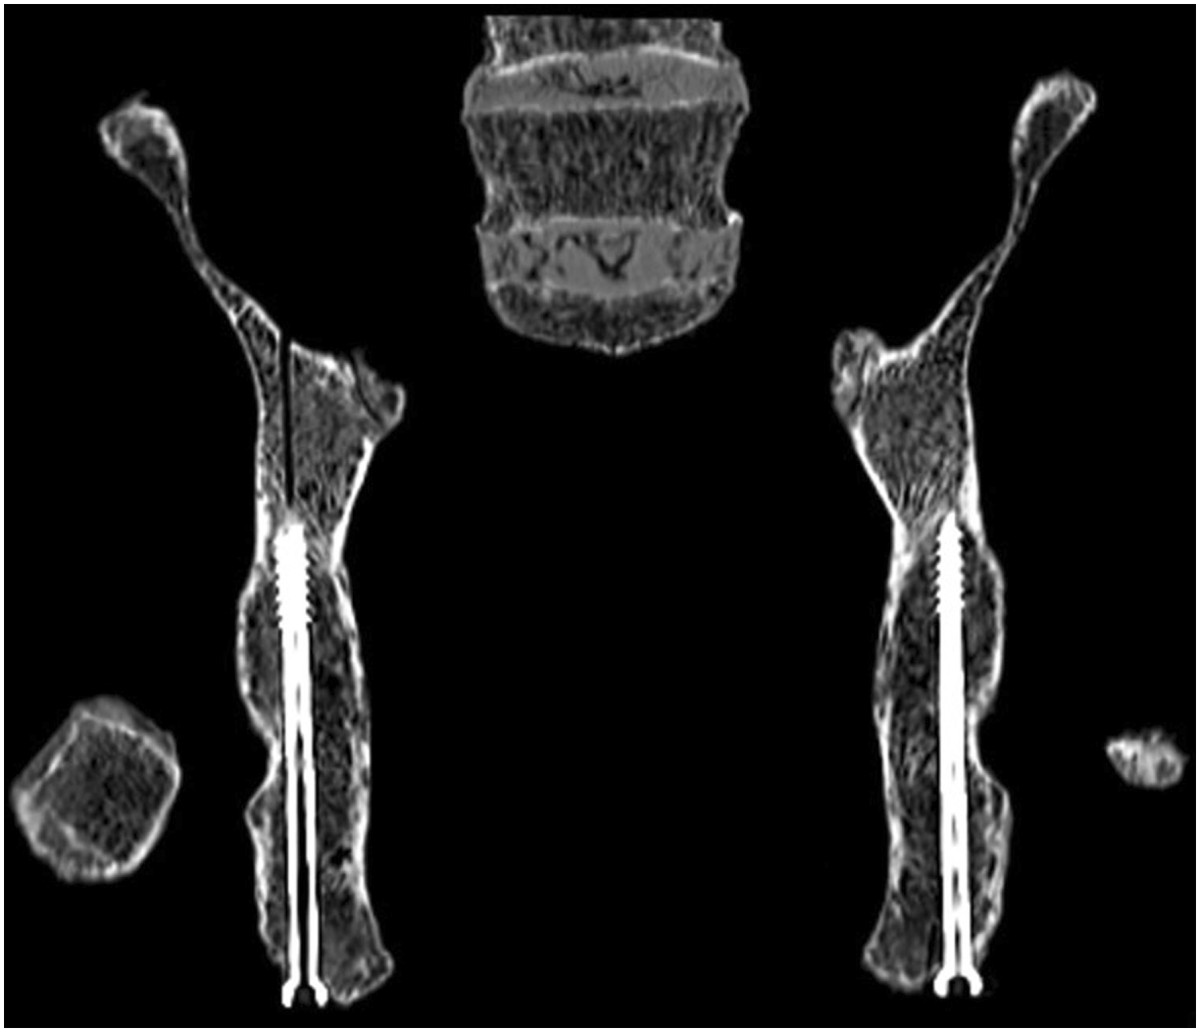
The oblique coronal reconstructed CT images shows that the full length of the lag screw is in the bony corridor of the posterior column, and the shortest distance between the posterior cortex and lag screw was at the levels of the greater and lesser sciatic notches.
